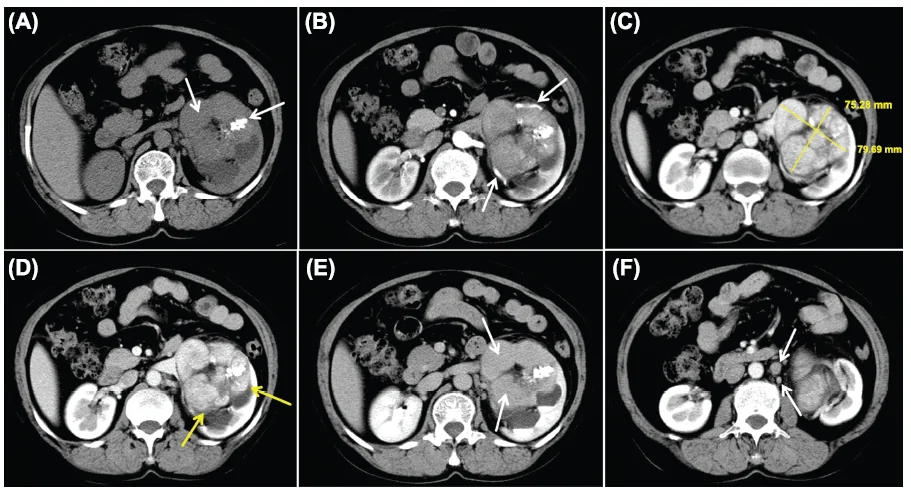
CT imaging features of left renal Castleman disease. (A) Non-contrast CT showing a soft tissue mass with nodular hyperdensities (white arrowheads). (B) Arterial phase demonstrating mild heterogeneous enhancement with displacement of the left renal artery (white arrowheads). (C) Venous phase showing progressive enhancement with well-defined margins measuring 80×75 mm. (D) Coronal reconstruction demonstrating pelvicalyceal compression with hydronephrosis (yellow arrowheads). (E) Delayed phase revealing contrast washout (white arrowheads). (F) Para-aortic lymphadenopathy at the level of the left renal hilum (white arrowheads).
